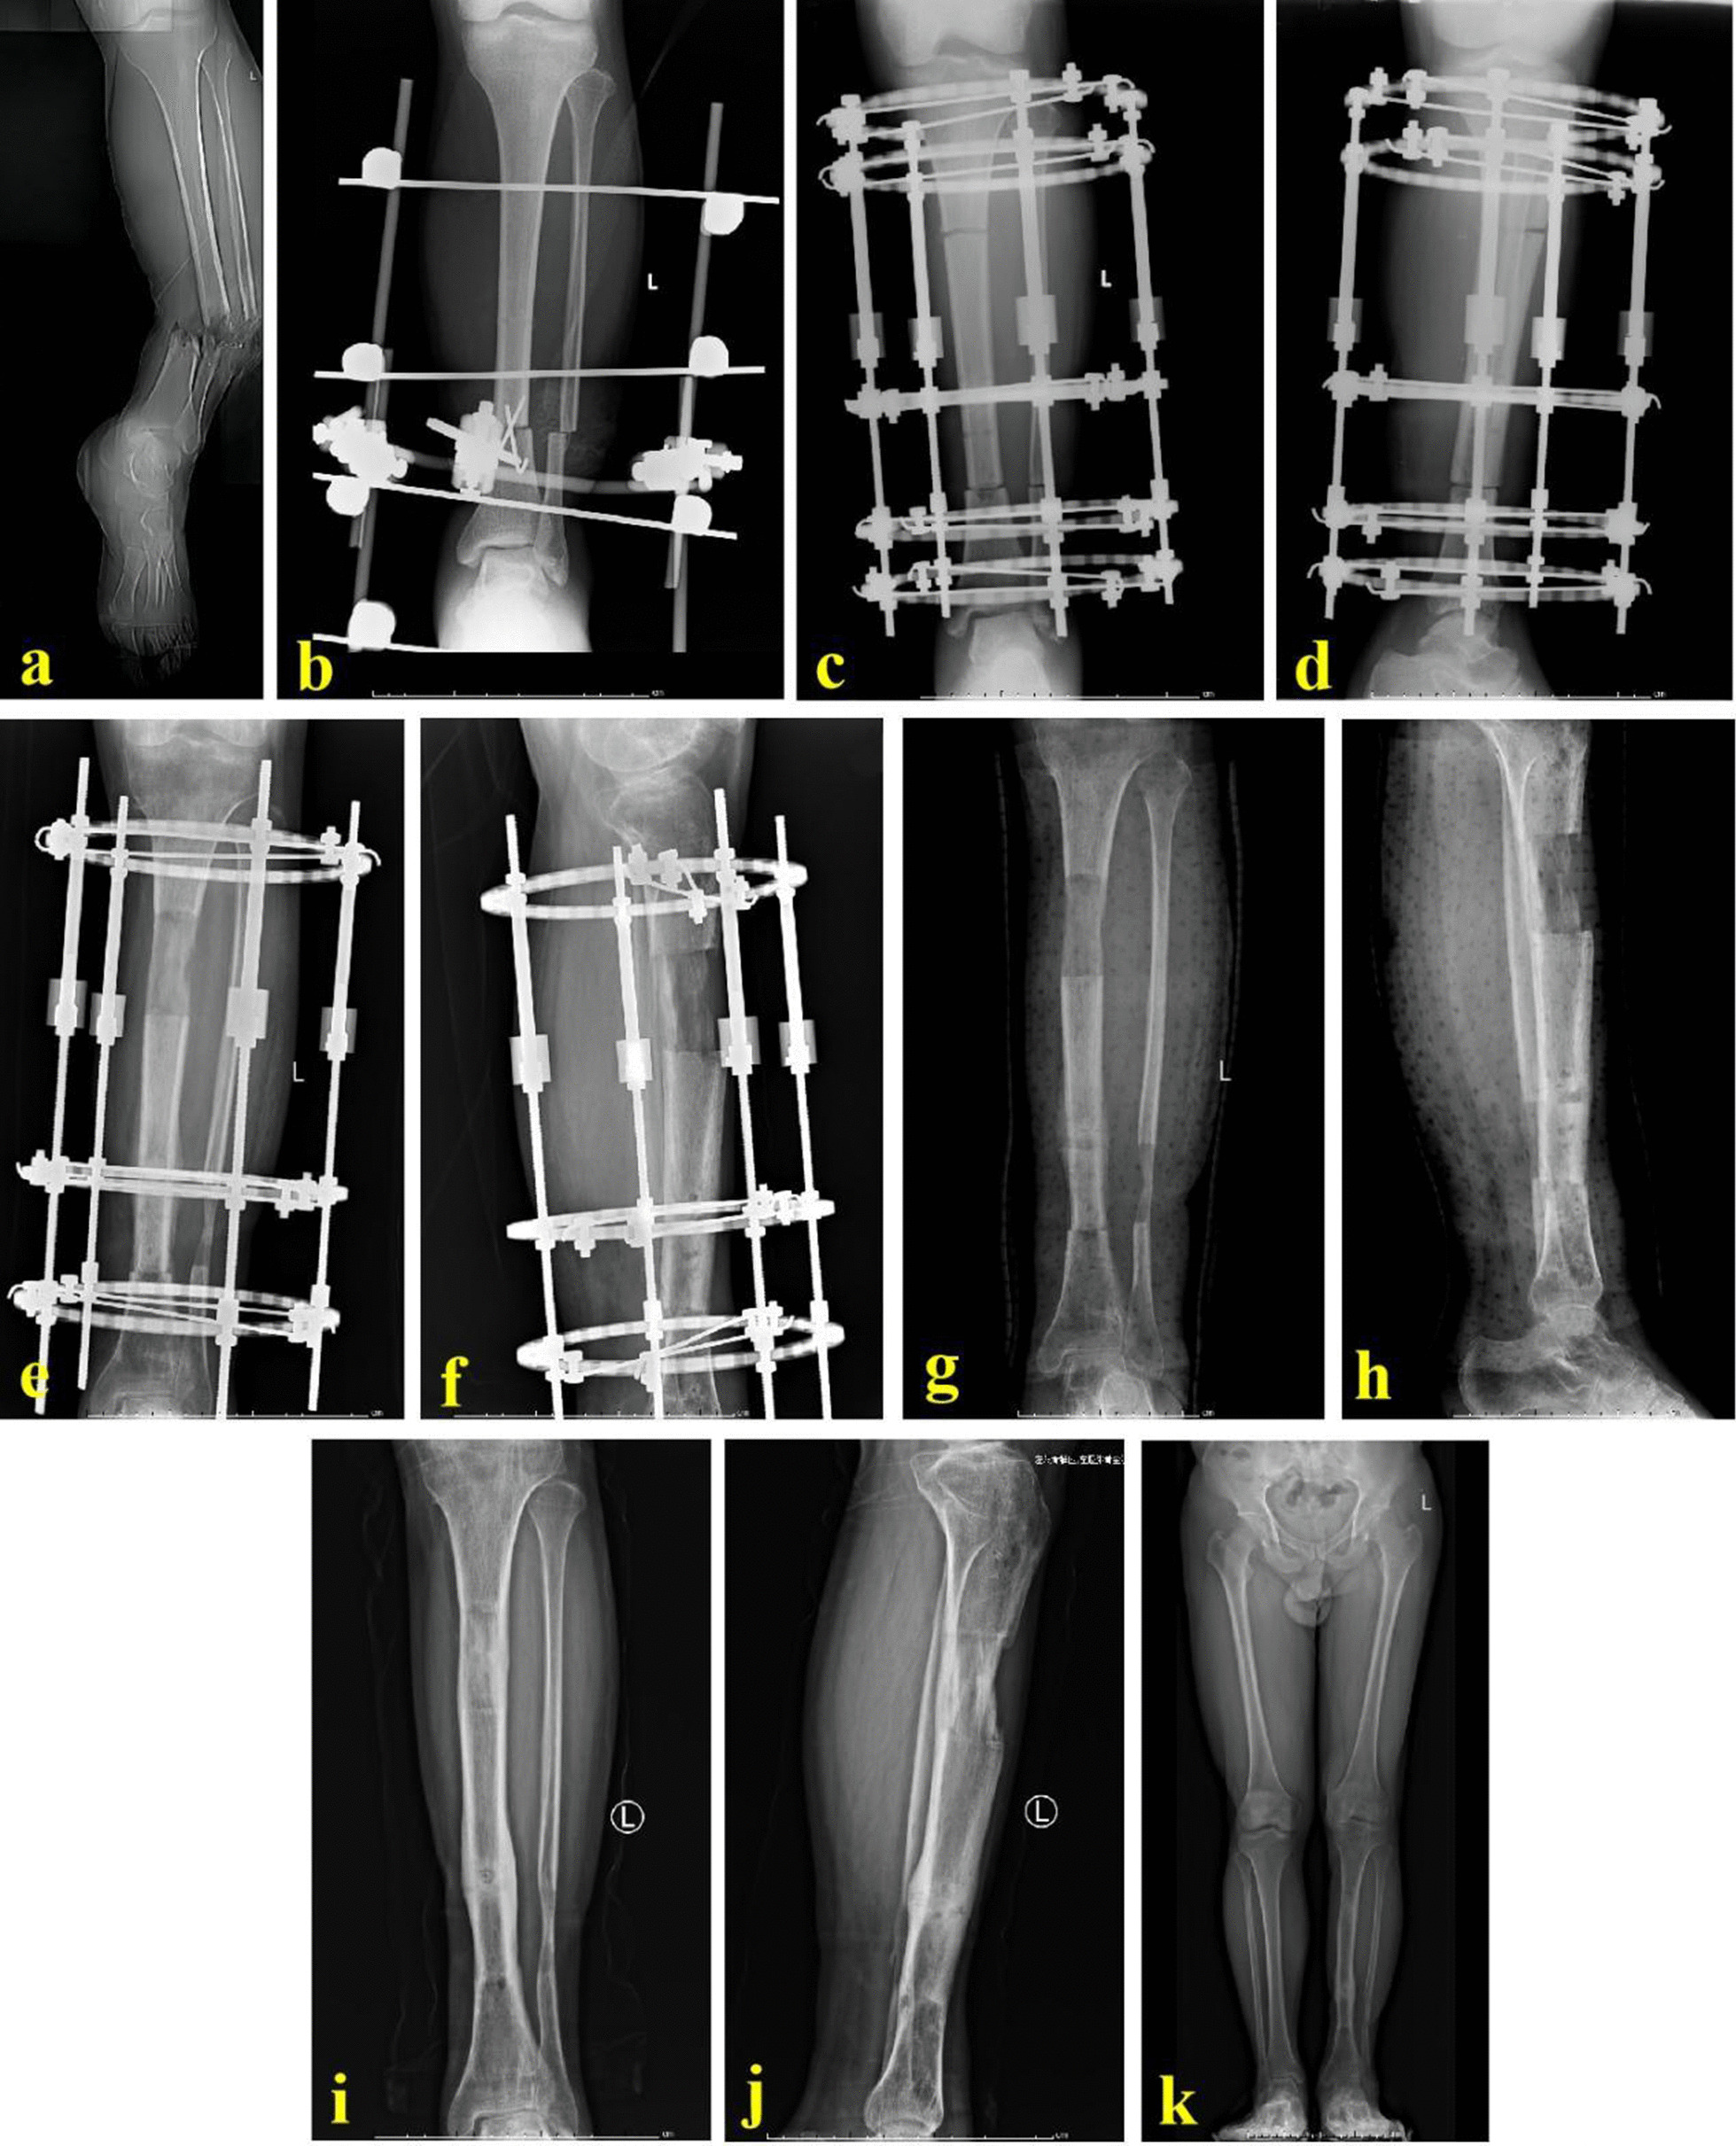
A 30-year-old male was successfully treated by ASR technique. a The patient suffered from a severe open fracture of the tibia; b after thorough debridement, acute shortening and temporary external fixation were performed. The tibial defects were 7.5 cm; c, d the Ilizarov annular lengthening frame was installed, and osteotomy was performed; e, f bone lengthening was successfully applied to restore the limb length; g, h X-ray images after removing the external frame; and i–k X-ray images at one year after removing the annular frame. ASR stands for acute shortening and re-lengthening
